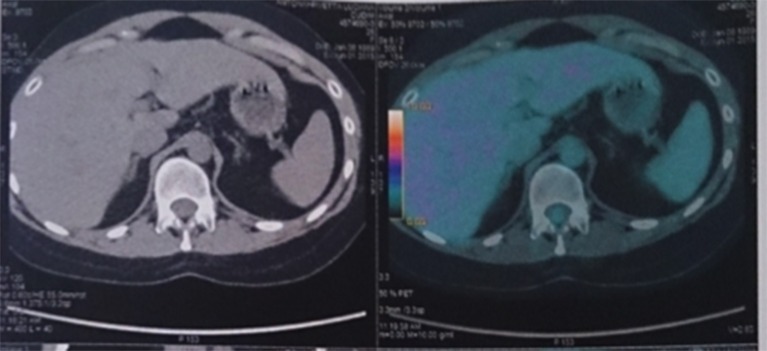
18F-FDG PET/CT scan showed no uptake in the adrenal glands.
